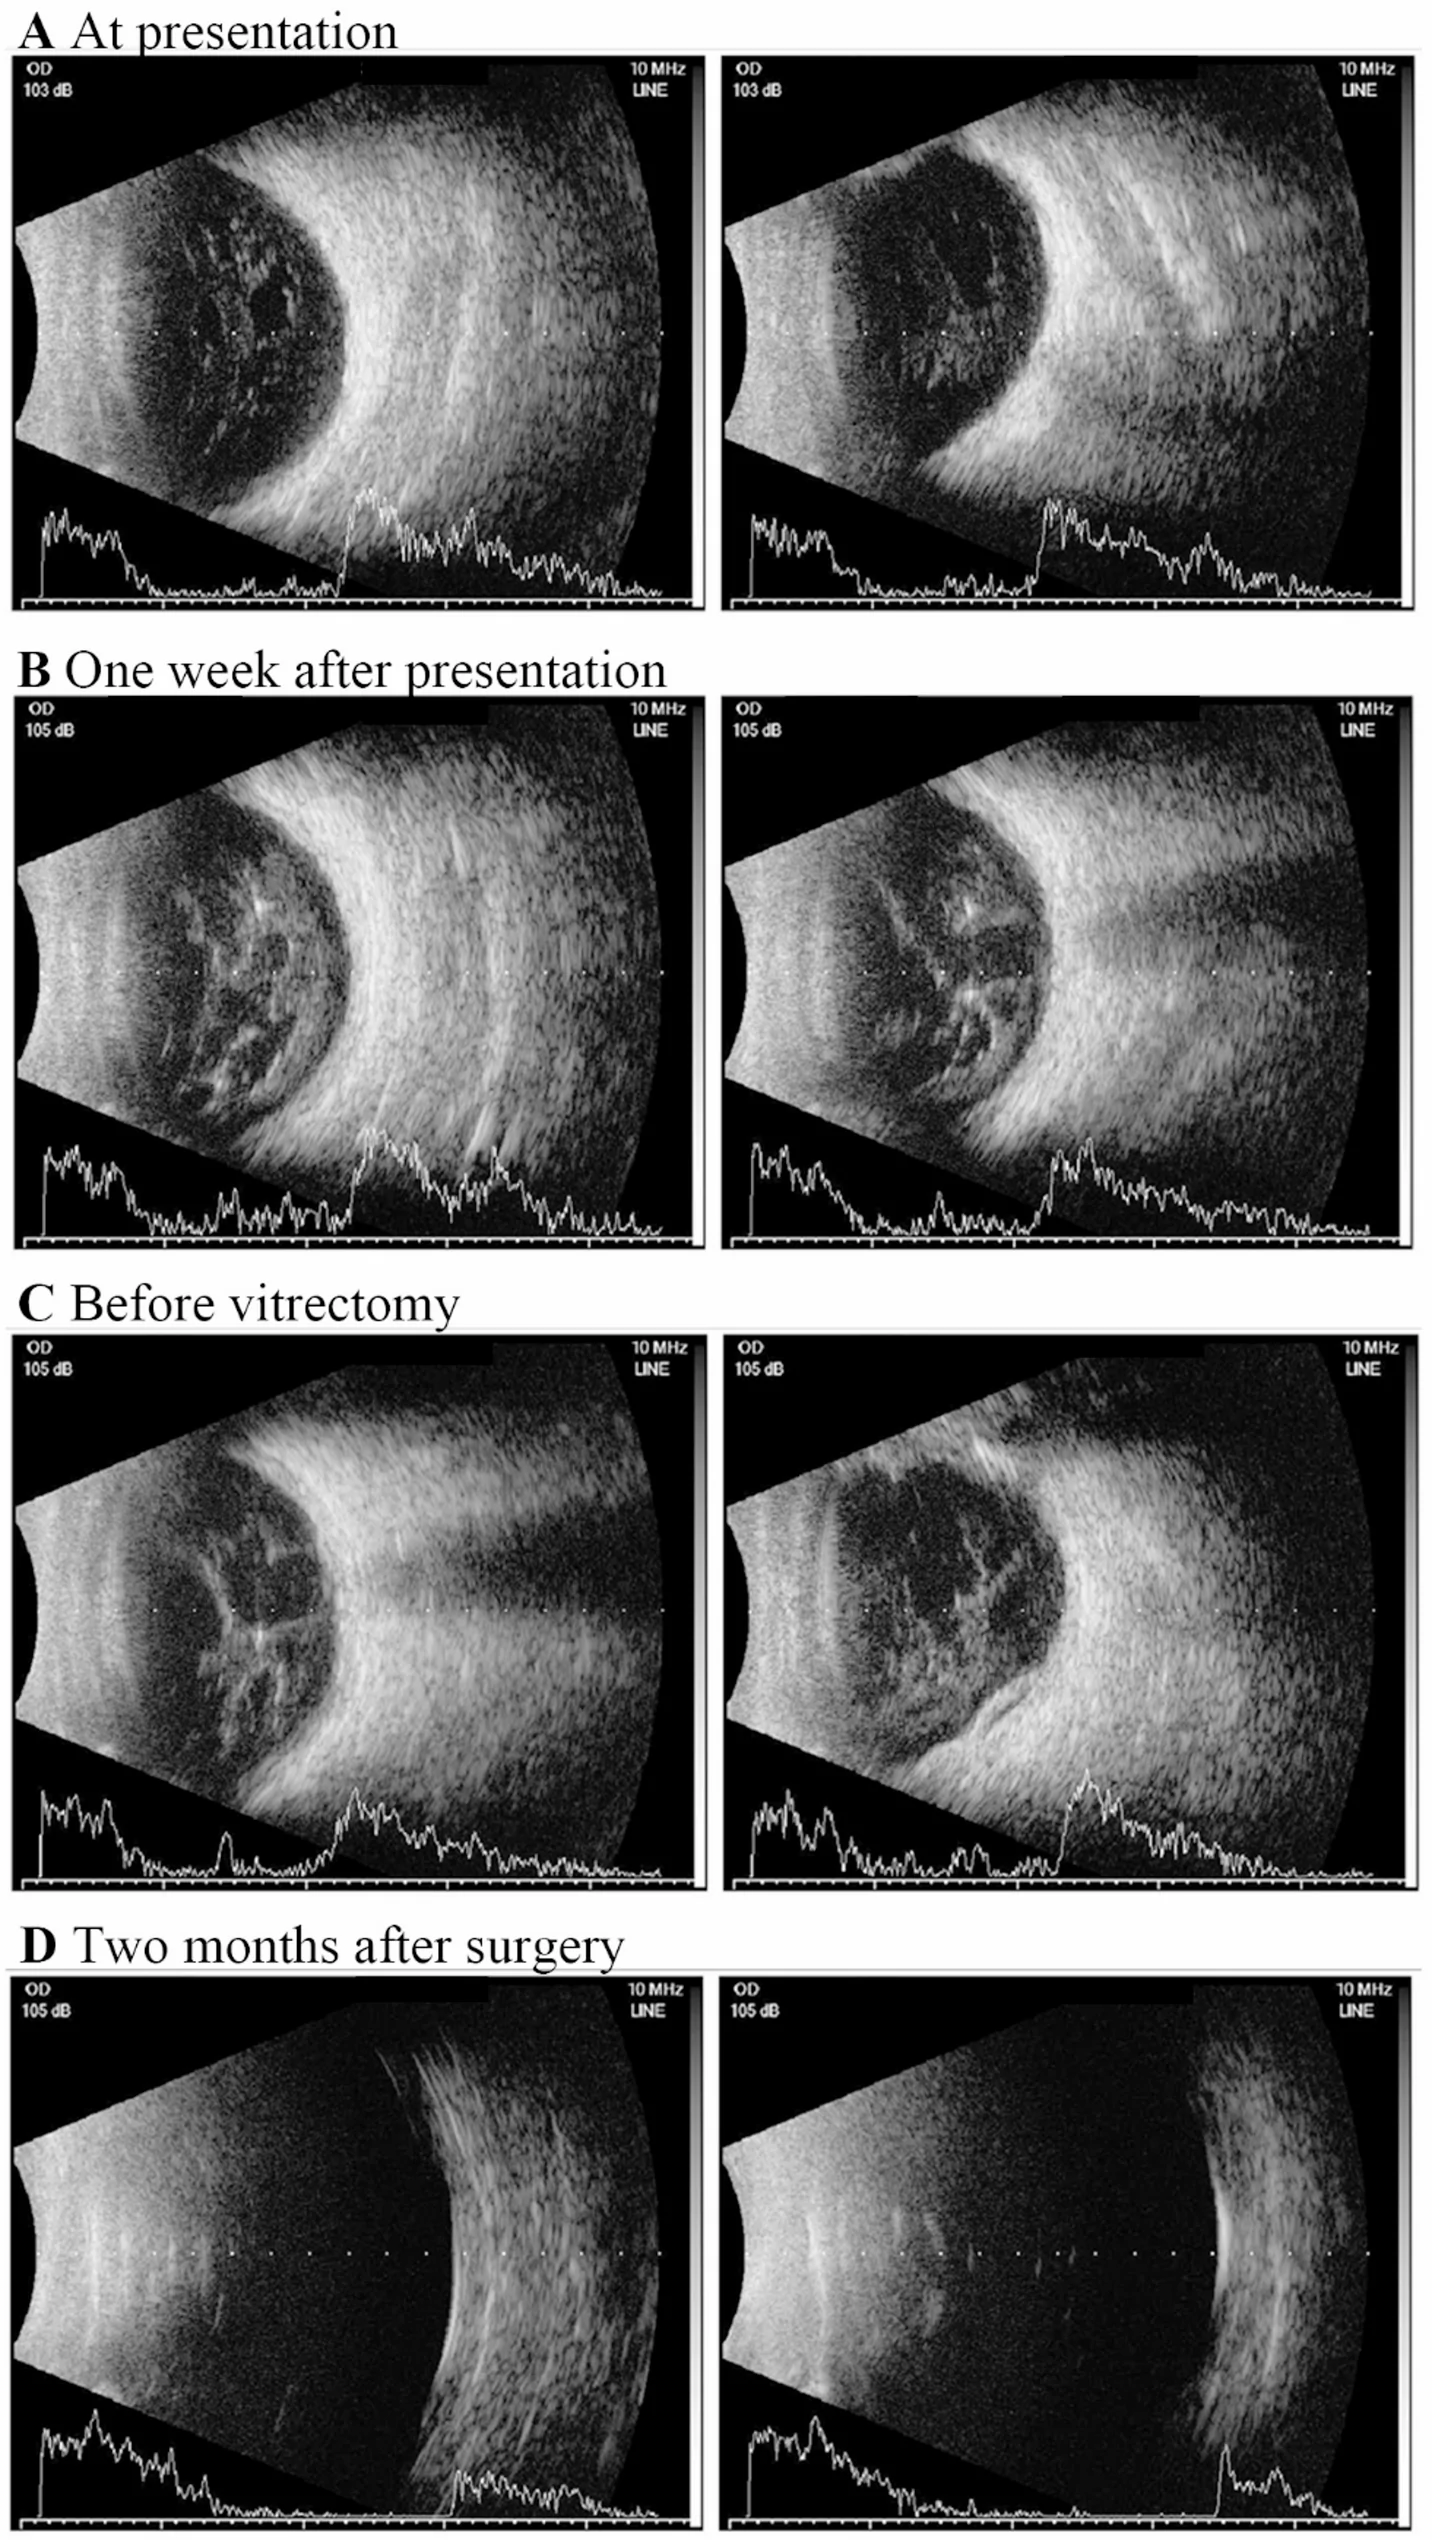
Serial B-scan ultrasonography of the right eye during follow-up. (A) At presentation, B-scan ultrasonography showed mild vitreous opacities, with no evident retinal detachment, choroidal detachment, or intraocular mass. (B) One week after presentation, repeat B-scan ultrasonography demonstrated increased vitreous echoes and debris. (C) Before vitrectomy, B-scan ultrasonography showed further progression, including dense vitreous opacities, localized shallow retinal detachment, and thickened scleral echoes. (D) Two months after surgery, B-scan ultrasonography showed typical postoperative features following vitrectomy and silicone oil tamponade
